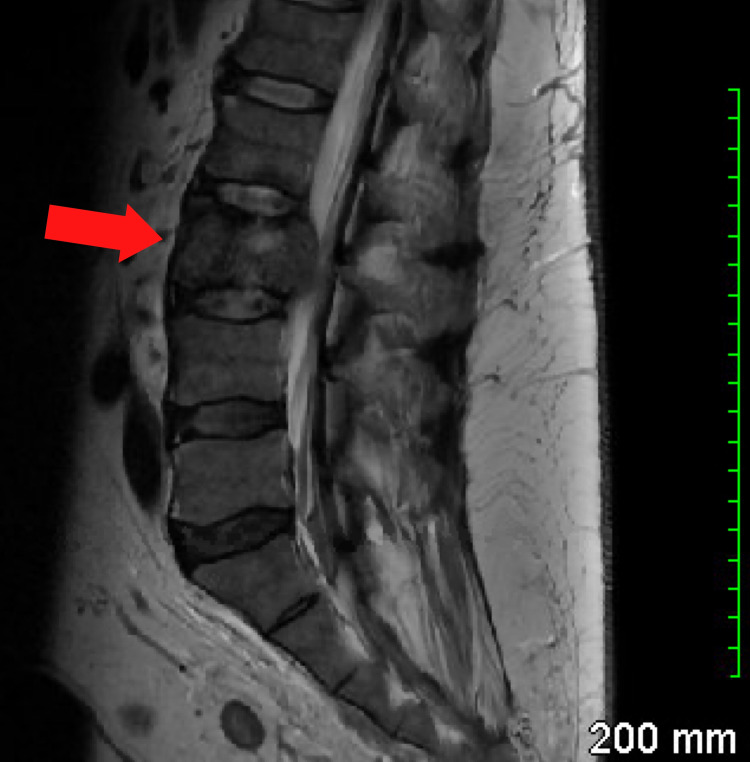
MRI lumbar spine (sagittal view). Aggressive marrow replacing a mass of the L3 vertebral body causing focal severe spinal canal narrowing. The red arrow indicates the mass effect in the vertebral body as a result of metastatic disease.
